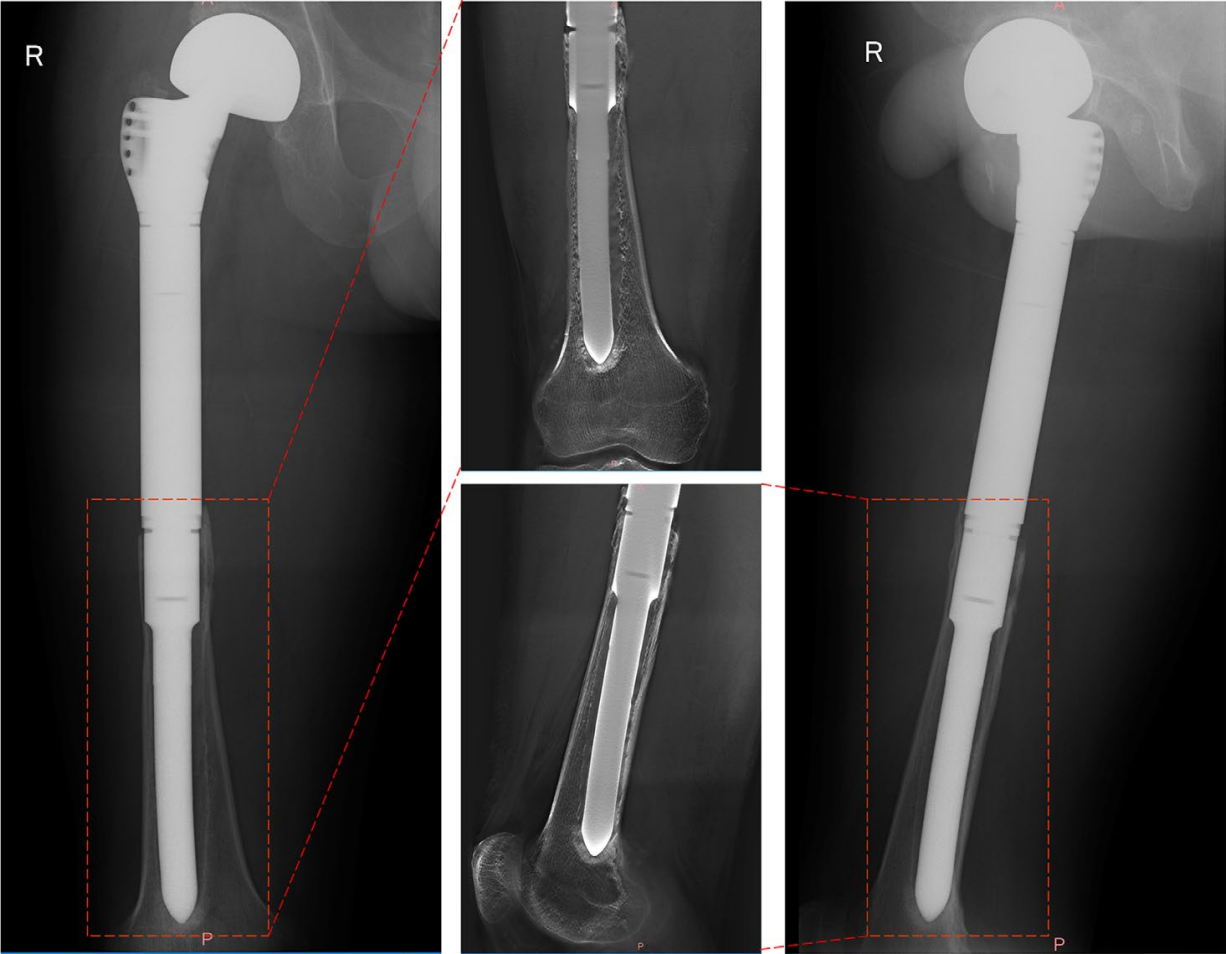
An aseptic loosening stem in a 25‐year‐old male patient, 18 months after implantation. Radiographic evaluations on anteroposterior and lateral views, combined with Tomosynthesis‐Shimadzu Metal Artifact Reduction Technology (T‐SMART).
